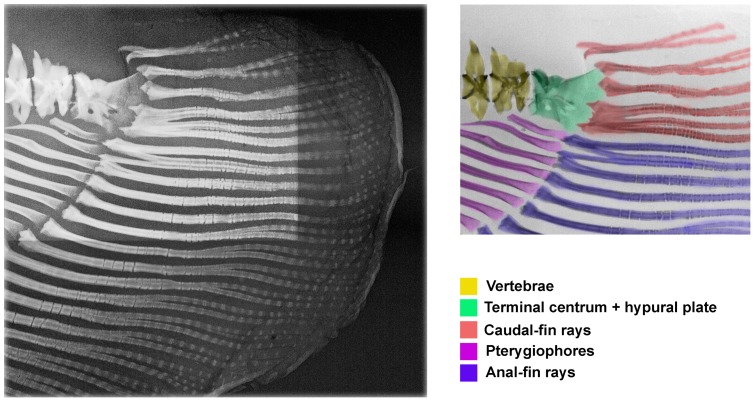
Radiographic image of caudal-fin region in *Electrophorus electricus,* USNM 225669, 500 mm TL. Color coding indicates components of caudal-fin skeleton, caudal-fin rays and posterior portion of anal fin.
